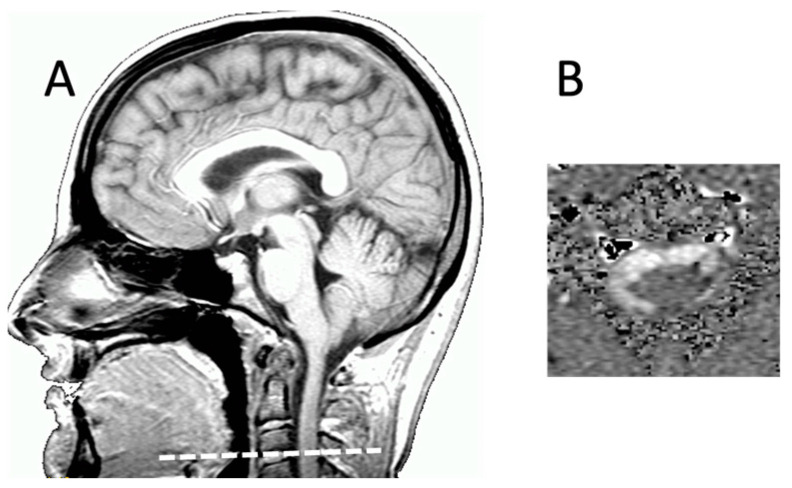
Slice plane position for acquisition of phase-contrast MRI for hydrodynamic evaluation. (A) Slice position on sagittal T1 acquisition through the C2C3 intervertebral disc and perpendicular to the assumed direction of circulation of CSF into the spinal subarachnoid spaces; (B) axial slice of phase-contrast MRI through the subarachnoid spaces at the level of the C2C3 intervertebral disc (encoding velocity = 5 cm/s) for analysis of CSF dynamics. This image represents 1 of the 32 phases studied during one cardiac cycle, with the subarachnoid spaces appearing like a white crown due to the caudocranial circulation of CSF. At other phases, it appears in black if CSF flows according a craniocaudal direction during CSF oscillation.
